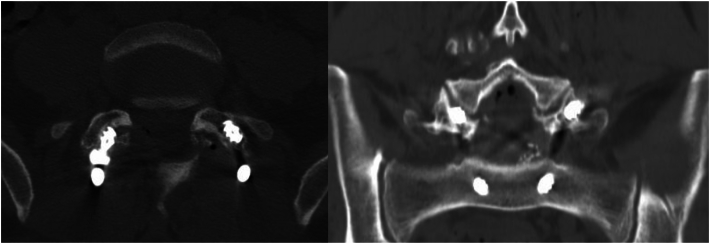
Axial and coronal slices of postoperative lumbo-sacral CT scan showing violation of the right L4-5 proximal facet joint.
